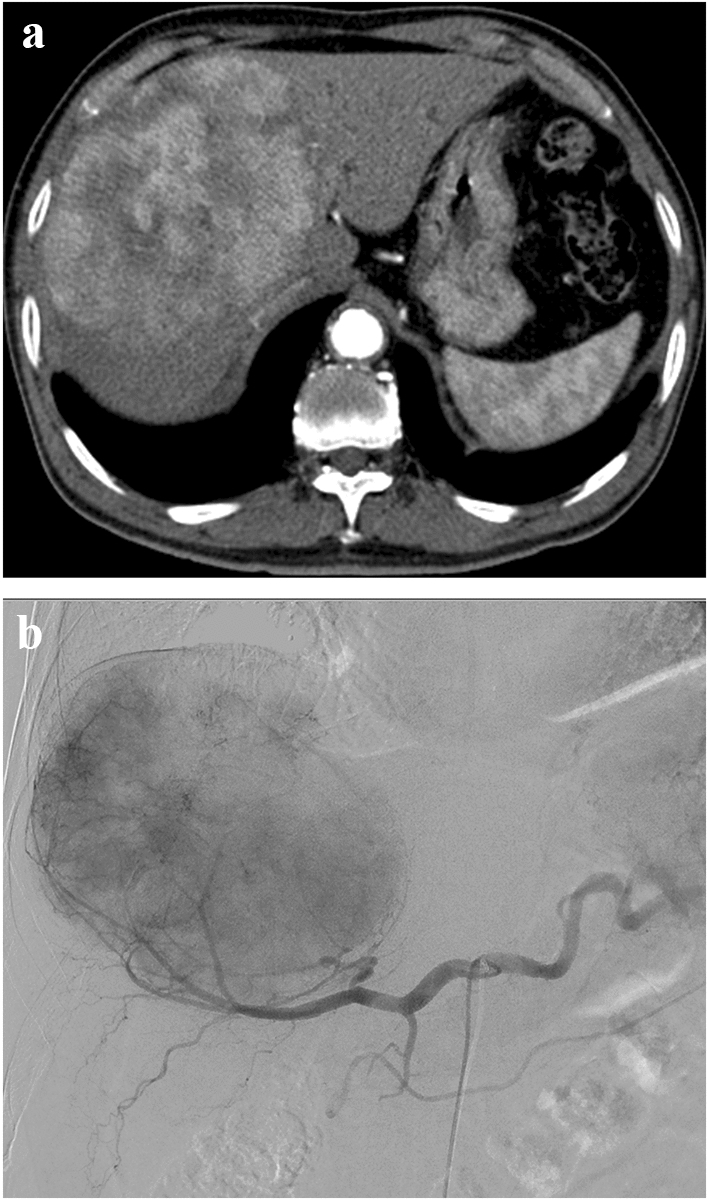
A 77 year old man with a hepatocellular carcinoma (size equal to 15 cm). (a,b) No dysmorphic intratumoral vessels were found on preprocedural liver computed tomography (CT) scans (a), and planning hepatic arteriography (b). In addition, there was no hepatic vein invasion or early hepatic vein enhancement on CT. The lung-shunt fraction was 0.79%.
